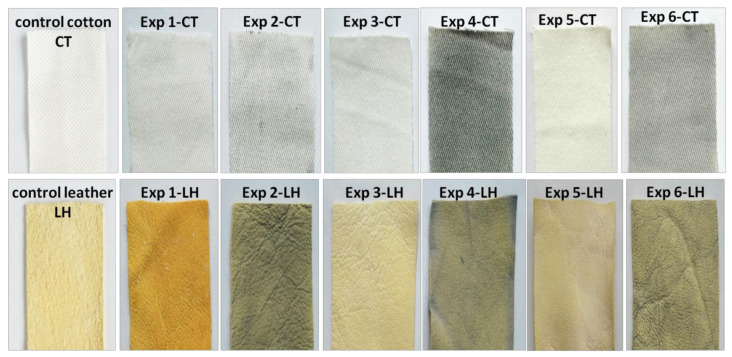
Digital photography of cotton (Exp 1-CT…Exp 6-CT) top row and leather (Exp 1-LH…Exp 6-LH) (bottom row) samples compared with control cotton (CT) and leather (LH) samples.
